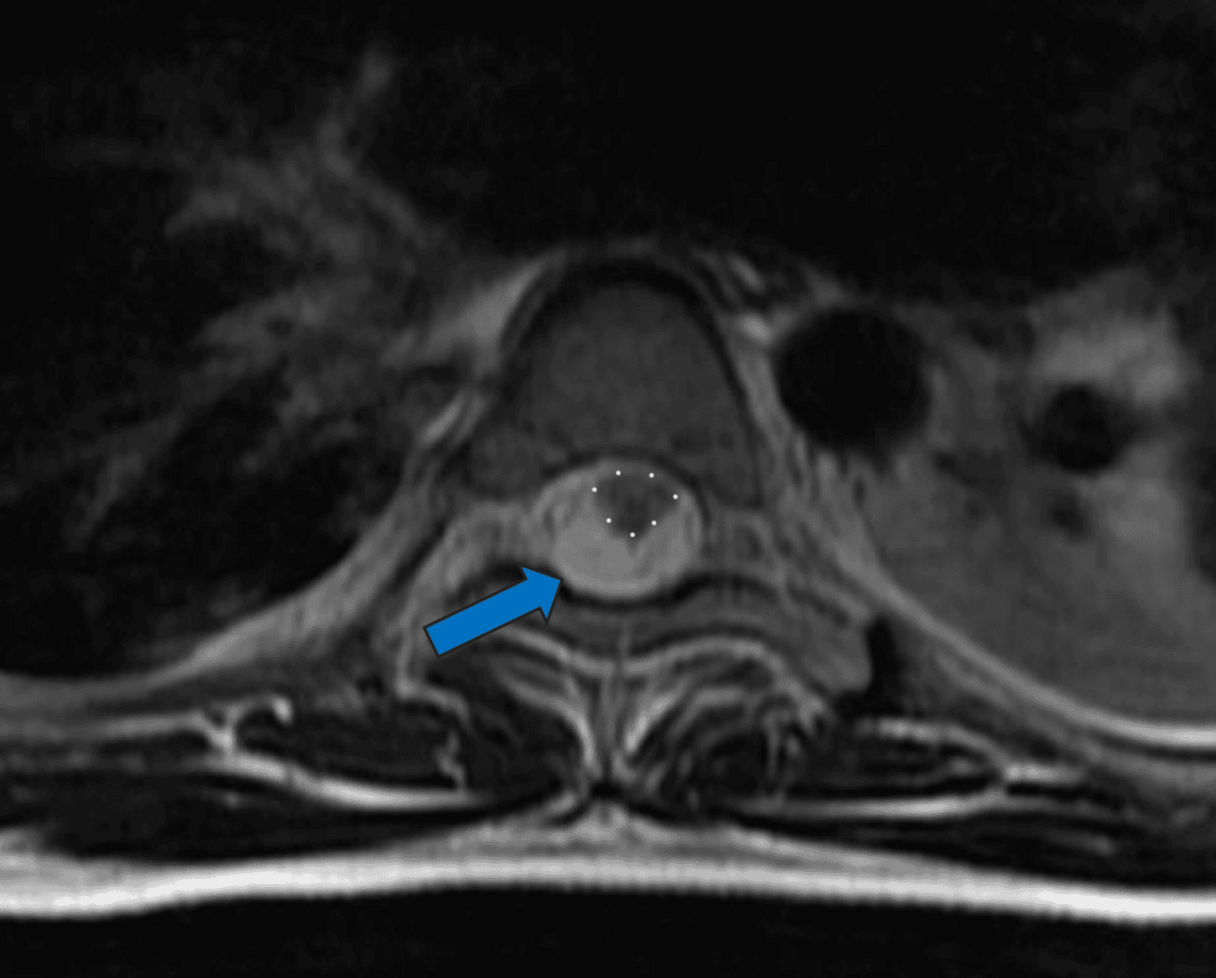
Axial T2 MRI showing metastasis (hyperintense area - blue arrow) compressing the thoracic spinal cord (hypointense area - outlined in white dots) again demonstrating disease progression with resulting lower and upper extremity weakness.
